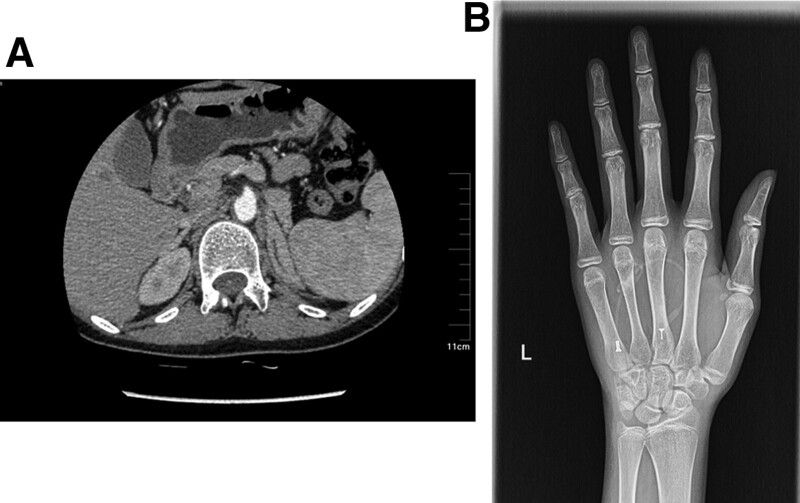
Clinical evaluations. (A) Adrenal CT showed a bilaterally longer and thicker gland. (B) X-ray imaging of carpal, phalanx and radioulnar bones.
